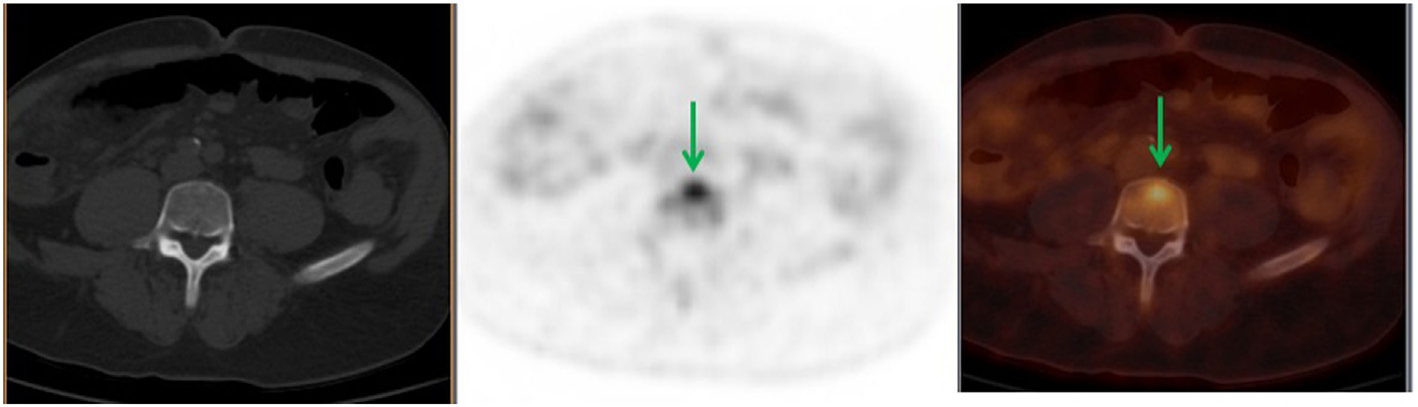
A 56-year-old male diagnosed with stage IIIC adenocarcinoma of the ascending colon status post right hemicolectomy. Follow-up, 18F-FDG PET demonstrated L5 FDG-avid lesion (green arrows) with no corresponding bone changes in the CT. This finding upstaged his cancer to stage IV. Patient received XRT and offered hospice.
